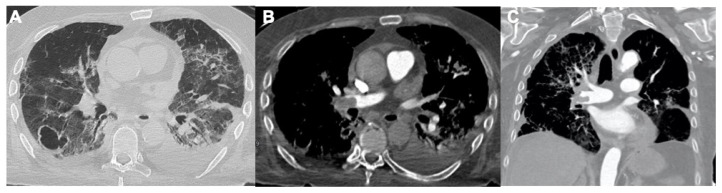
A 67-year-old male with acute pulmonary embolism related to COVID-19 infection. (A) Cavitation in right lower lobe associated with pericardial and pleural effusions. (B) Axial view of computed tomography pulmonary angiogram (CTPA) shows an acute filling defect in right main pulmonary artery. (C) Coronal views of same patient.
